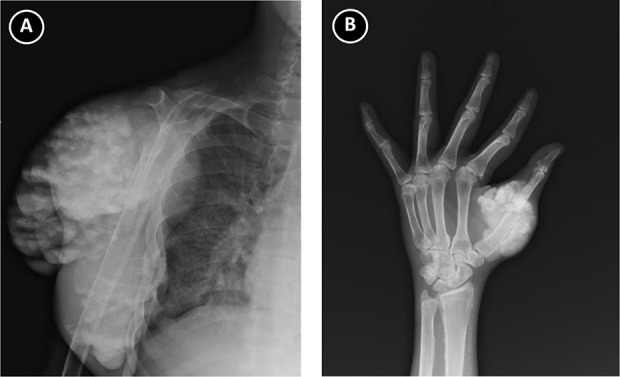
Plain radiograph of the patient. (A) Radiograph of right shoulder showed amorphous, multilobulated, and cystic calfication in periarticular location. (B) Radiograph of left hand showed amorphous, multilobulated calcification around promixal interphalangeal joints of the first finger.
